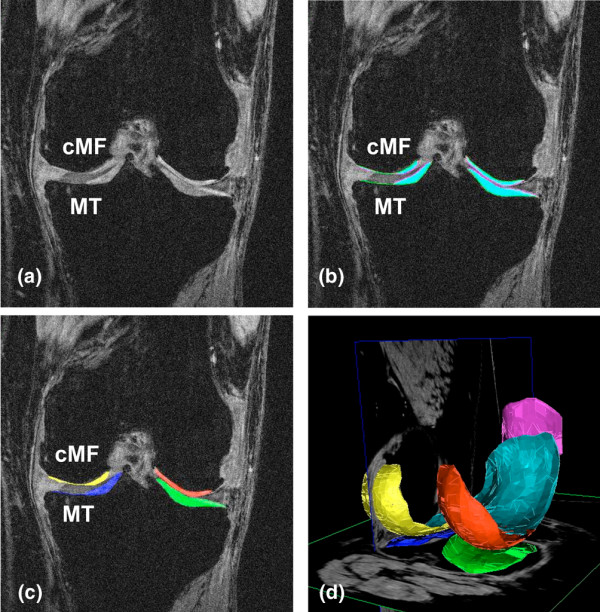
Knee magnetic resonance image obtained with fast low angle shot (FLASH) sequences with water excitation. (a) Double oblique coronal image showing the regions of interest used in the current analysis. (b) Same coronal image with the total area of subchondral bone (tAB) being segmented in green, the area of the cartilage surface (AC) in magenta, and the filling between the two surfaces in turquoise. Note the denuded area (tAB not covered by AC) in the medial tibia and the medial femur. (c) Same coronal image with the medial tibial (MT) cartilage marked (segmented) blue, weight-bearing medial femoral cartilage (cMF) marked yellow, the lateral tibial cartilage marked green, and the lateral weight-bearing femoral cartilage marked red. (d) 3D reconstructions of knee cartilage plates from a sagittal data set in a different person: The femoro-tibial cartilages are labeled with the same colors as in (c), the patellar cartilage is labeled magenta and the trochlear (femoral) cartilage in turquoise.
